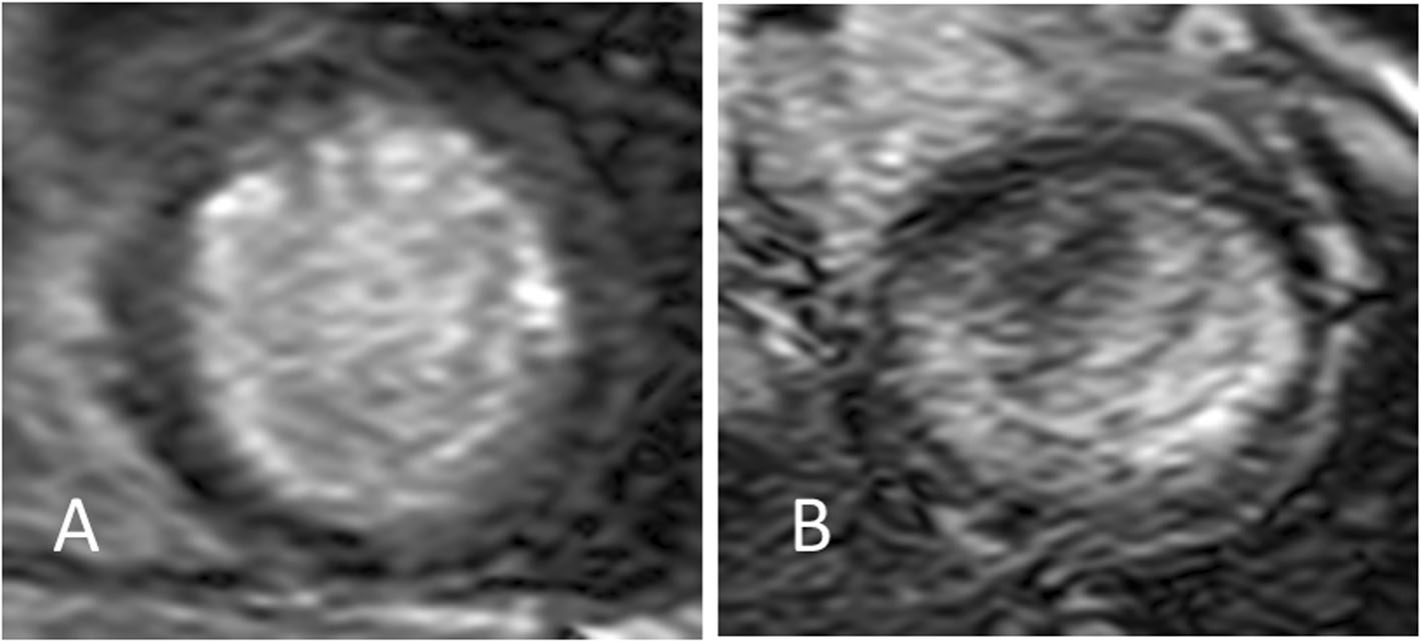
Late gadolinium-enhanced MRI. a. In Case 1, subendocardial lesions were detected in the anterolateral area. b. In Case 2, a subendocardial laminar lesion was detected in the lateral area
